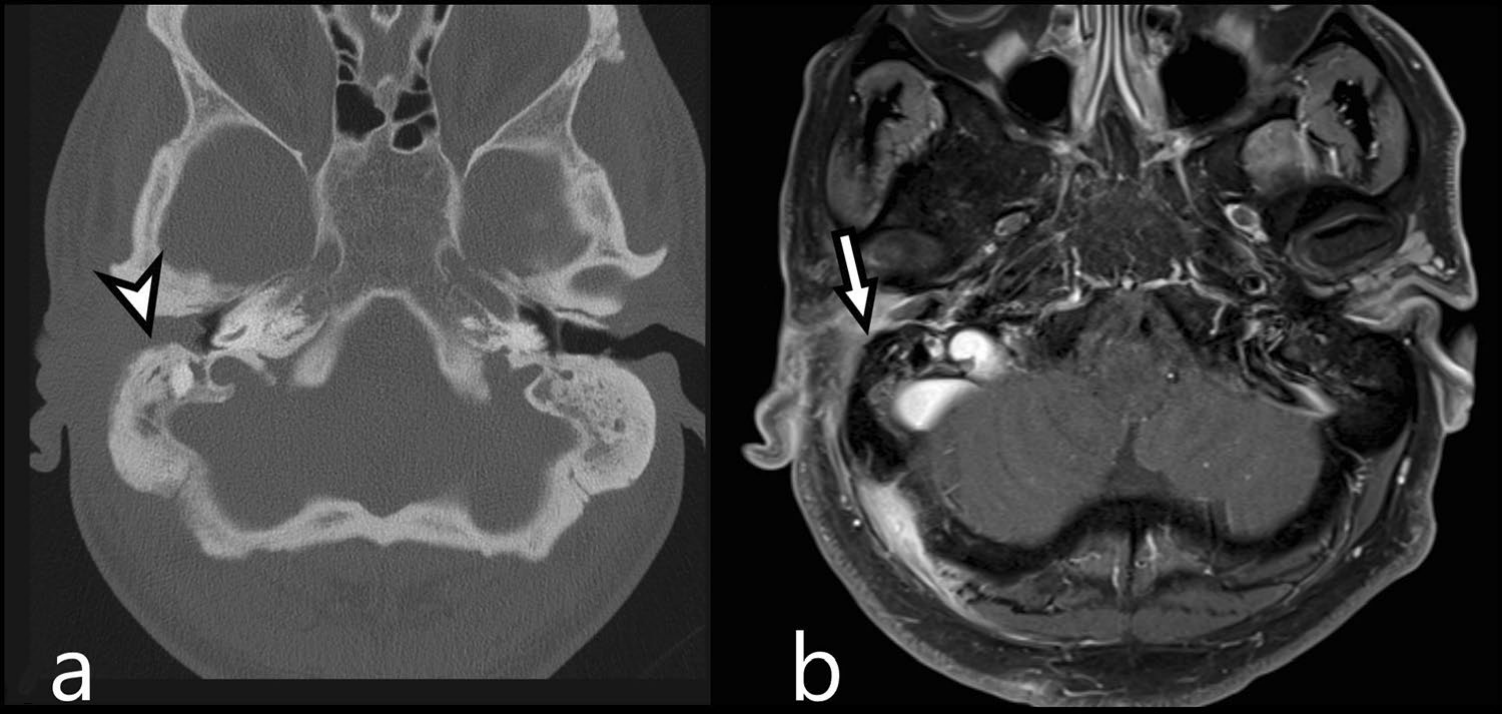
Example of a posterior spreading pattern: a 46-year-old male presented with right sided otalgia, jaw pain and N.VII. paralysis. The CT shows subtle destruction of the mastoid part of the temporal bone (a; arrow head). MR T1-fs gado sequences show enhancement of the external ear but no signal abnormalities of the mastoid (b; arrow). The increased signal intensity in the venous sinus is due to slow flow
